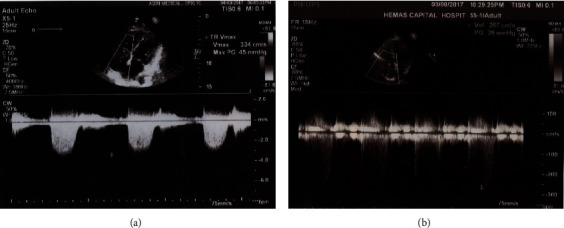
Transthoracic echocardiography demonstrating pulmonary artery pressures before (a) and after (b) the treatment of thyrotoxicosis.
